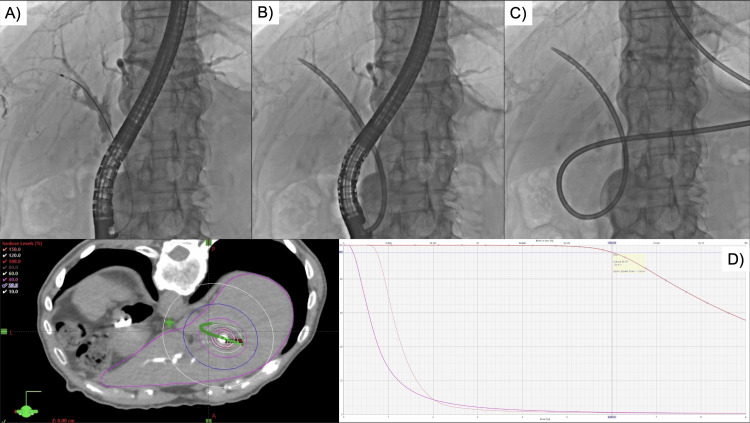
High-dose-rate brachytherapy under endoscopic retrograde cholangiopancreatography for Intraductal papillary neoplasm of the common bile duct-right intrahepatic bile duct A) Performing endoscopic retrograde cholgiopancreaticography (ERCP) and confirming lesion with cholangiography (Step 1). B) 10 Fr ENBD tube implanted via guidewire (Step 2). C) Confirmation that the source can pass through the brachytherapy applicator (Step 3). D) Treatment planning computed tomography (CT) is performed in the prone position to create a treatment plan (Step 4). The dose-volume histogram for the initial treatment plan shows 6.5 Gy for 90% of Target (red), 3.0 Gy for D1cc in the duodenum (pink), and a normal mean liver dose (blue) of 1.0 Gy.
